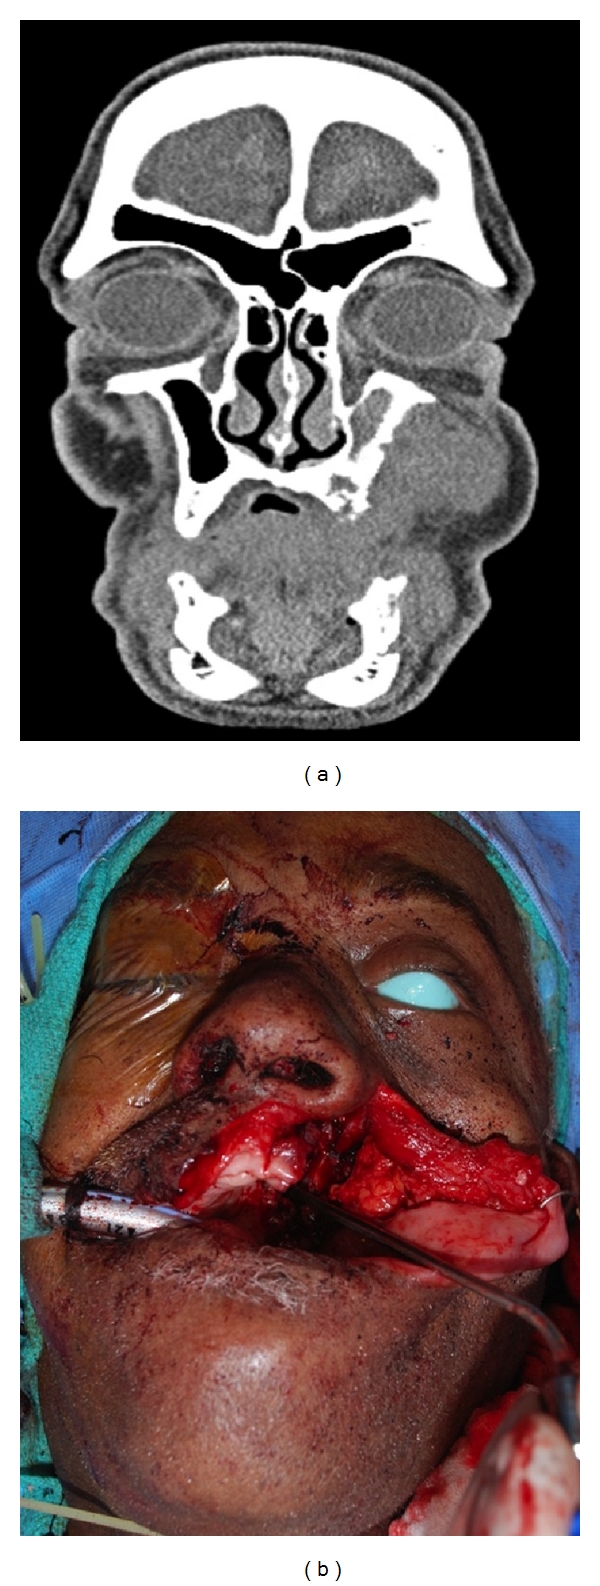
Preoperative coronal CT scan demonstrating invasive midface lesion and resultant defect after extirpation.
